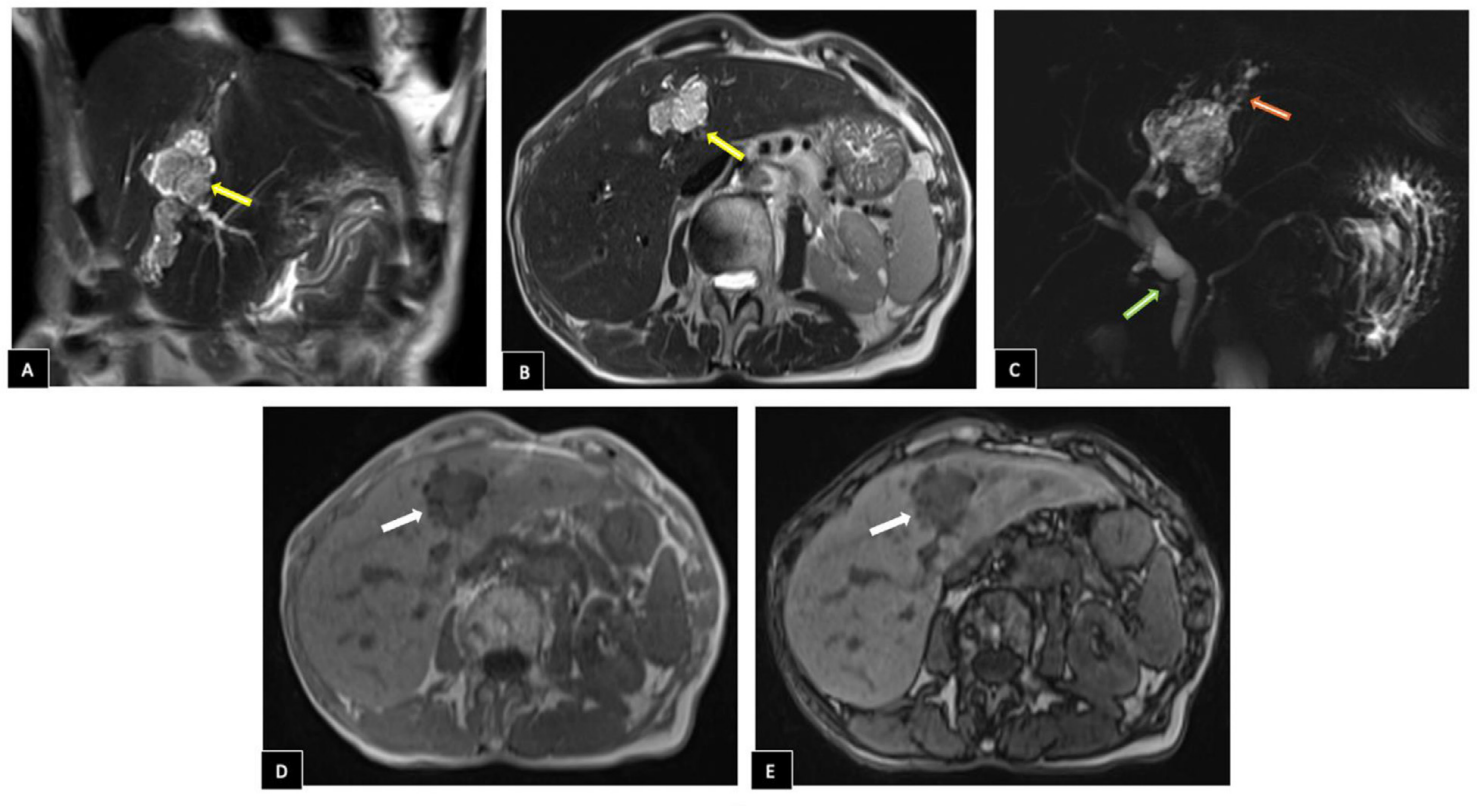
Liver MRI Imaging Findings. (A, B) Coronal and axial T2 images revealing an intraductal mass between segments IV and III with lobulated contours heterogeneous hyperintense, extending within the biliary duct of segment III (yellow arrows). (C) Coronal conventional T2-weighted MR cholangiography shows mild upstream intrahepatic segmental bile duct dilatation (red arrow ) as well as moderate dilatation of extrahepatic bile ducts (green arrow). (D) In- and (e) opposed-phase T1-weighted imaging indicate absence of intralesional fat (white arrows).
